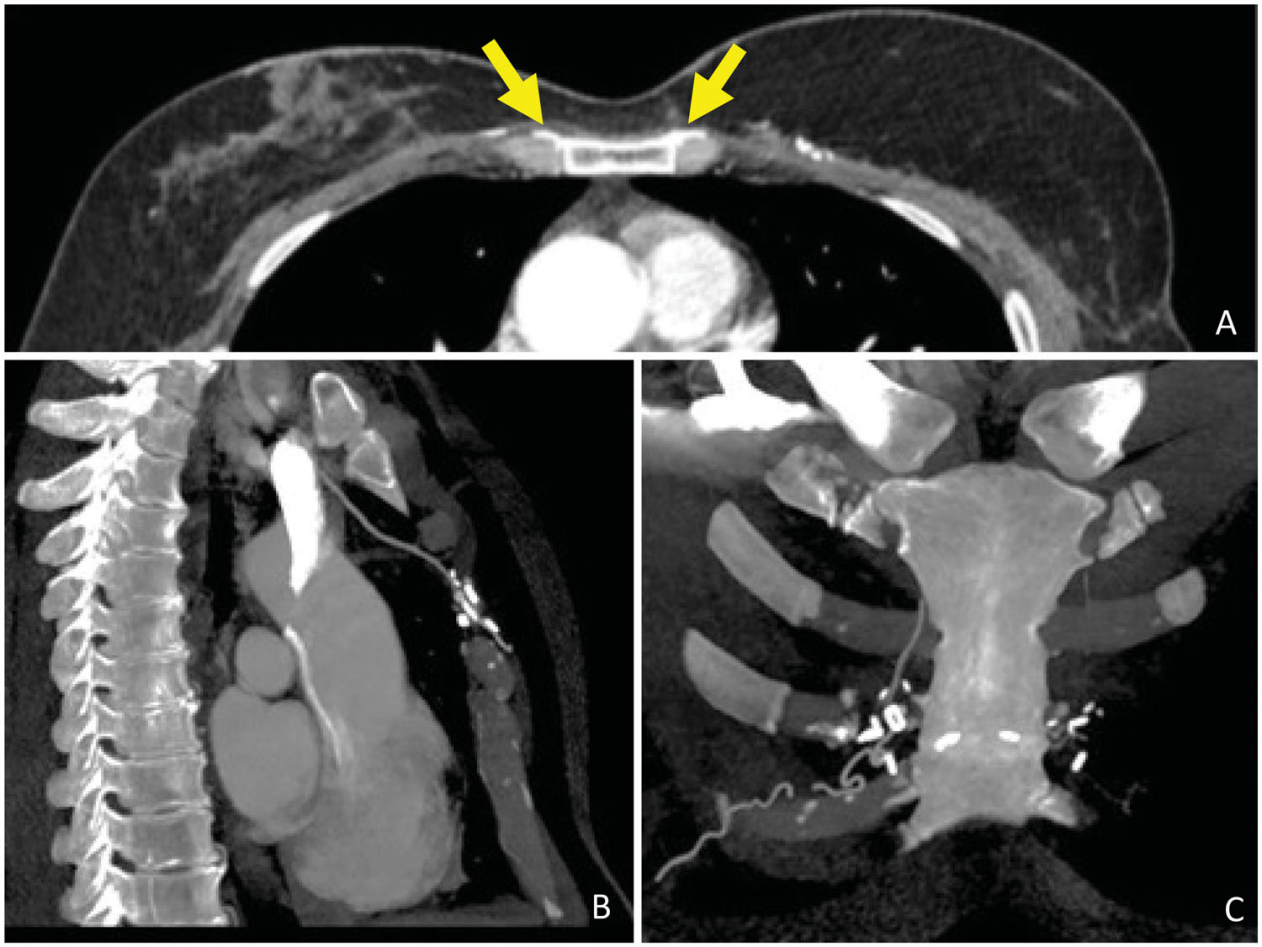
Postoperative CT scan. (A) Axial view, contrast enhancing shows DIEP vessels crossing the sternum (yellow arrows). (B) Sagittal view, IM vessels anastomosed to presternal vessels. (C) Coronal view, microvascular staples highlight the DIEP vessels path through the midline.
